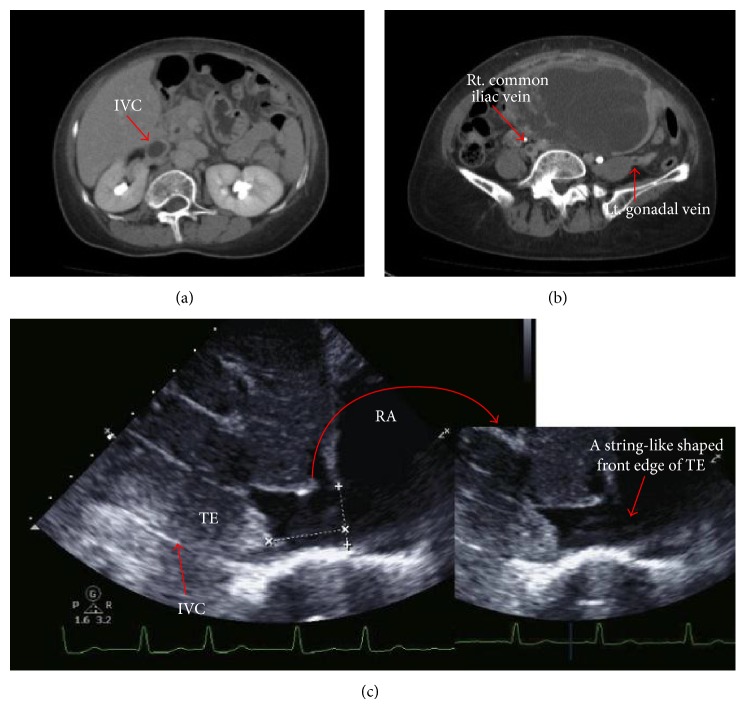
(a, b) Axial computed tomography revealed tumor emboli extending from the uterus to the inferior vena cava. (b) Right common iliac and left gonadal veins. (c) Cardiac ultrasonography revealed a string-like front edge of the tumor embolism inside the right atrium. IVC, inferior vena cava; TE, tumor embolism; RA, right atrium.
